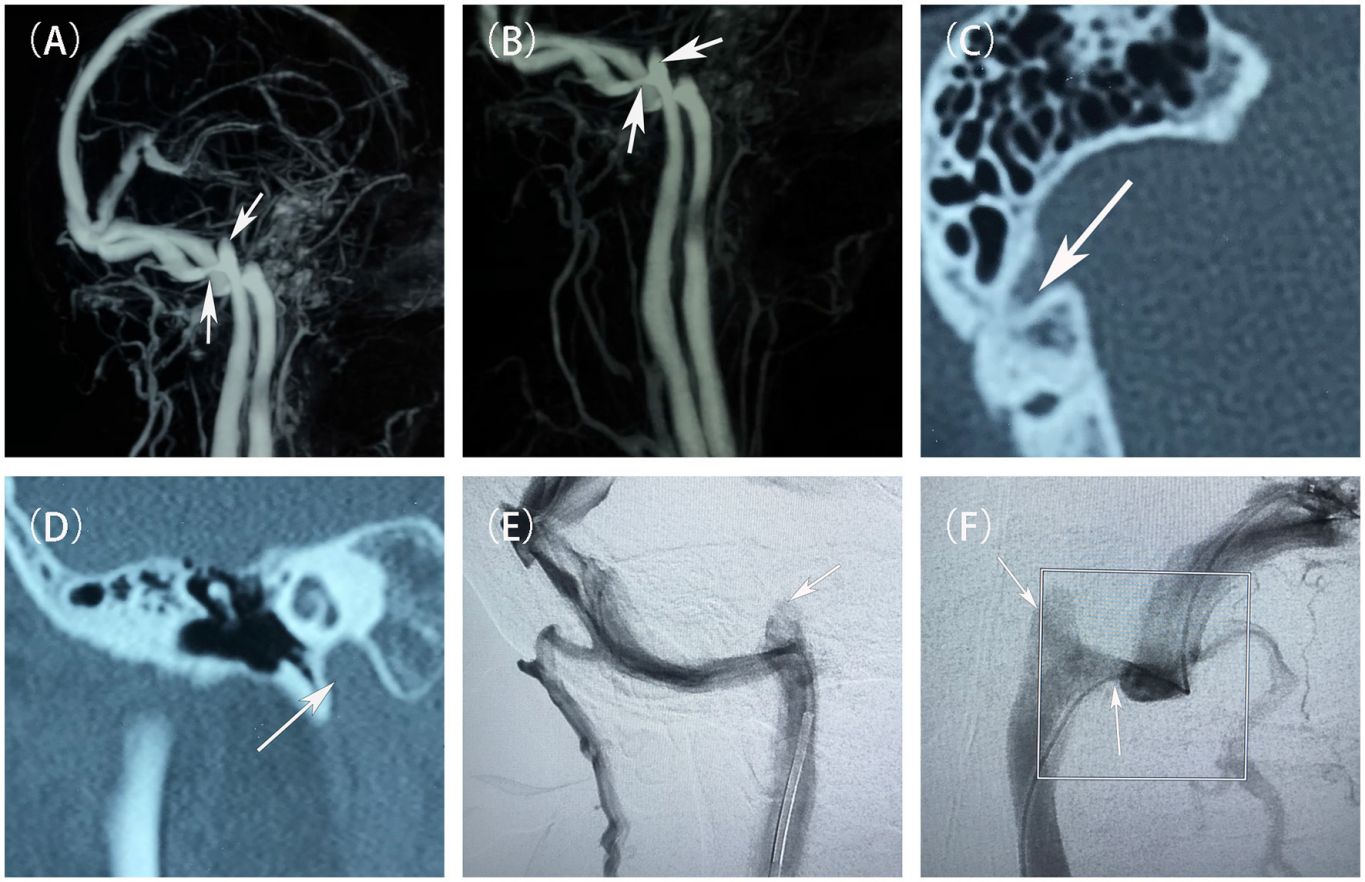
Preoperative evaluation and diagnostic imaging of the patient. (A, B) Pre-treatment MRV scans showed the stenosis at the junction of the right sigmoid sinus and the internal jugular vein, and a high jugular bulb (white arrow). (C) Pre-treatment non-contrast CT scans showed a branch of the mastoid vein emanating from the right sigmoid sinus. (D) Pre-treatment non-contrast CT scans demonstrated the right high jugular vein bulb invaded the inner ear structures. (E, F) Pre-treatment DSAs showed that a narrowing of the sigmoid sinus at its junction with the internal jugular vein, and a high jugular bulb (white arrow).
